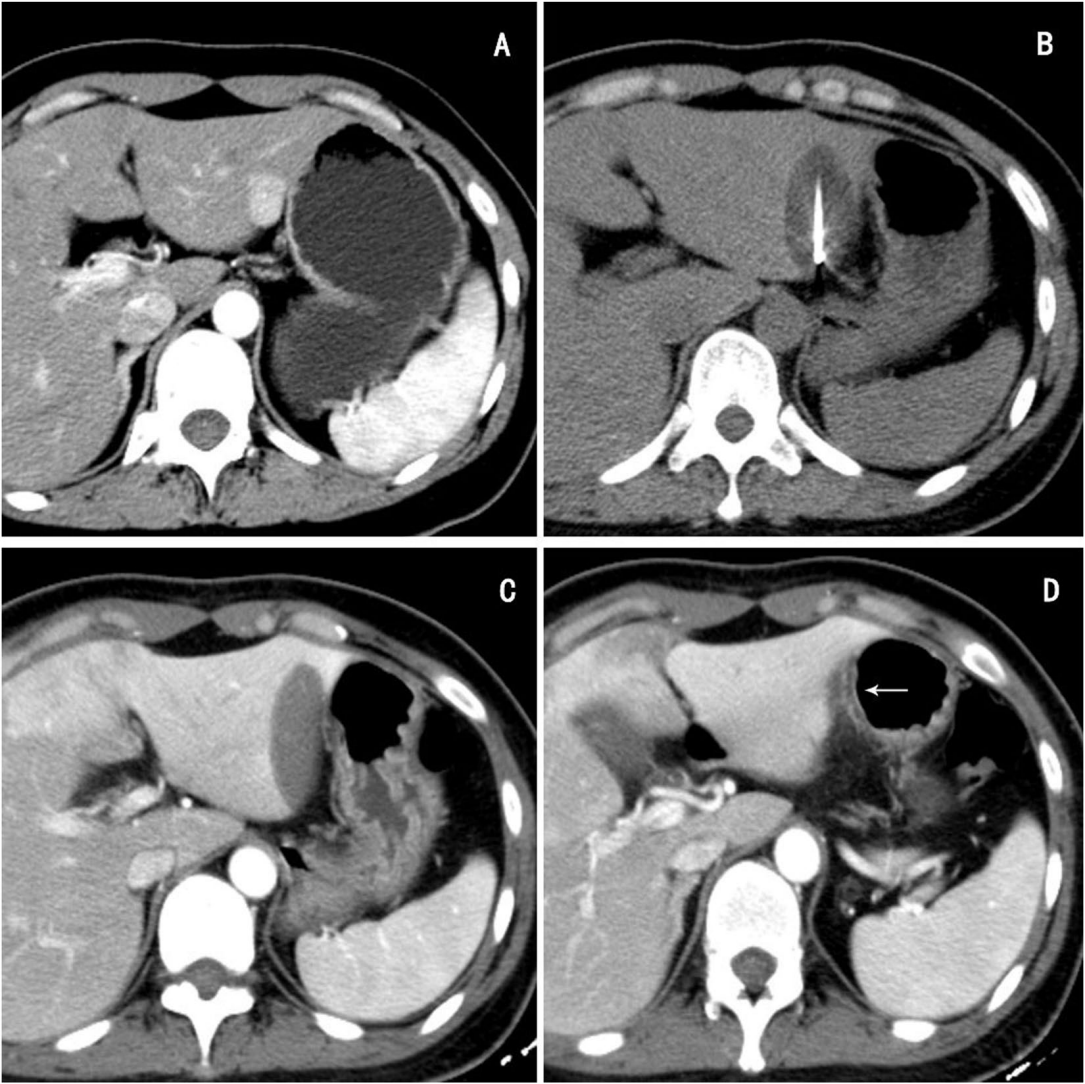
A 34 year old female; (A) A nodule in left lobe was detected accidentally during a routine check-up. (B) Cryoablation was applied in liver lesion under CT guidance without induction of artificial ascites. (C) and (D) The CT re-examination within three days showed complete ablation of the lesion (C) slight thickening, and edema of the gastric wall adjacent to the lesion (D).
